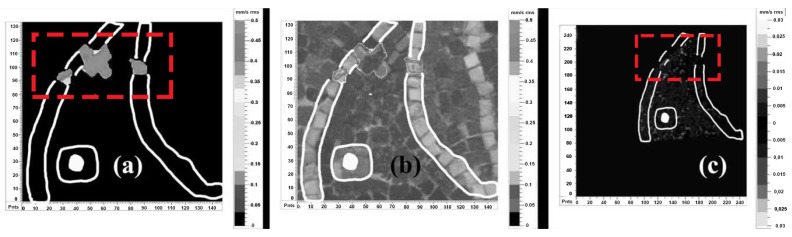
Scanning laser doppler vibrometry (SLDV) results in a custom-built mosaic model. Vibration maps of sample mosaic at 1525 Hz (a) a grayscale image representing the vibrational amplitude before restoration, the areas of detached tesserae show higher amplitude, corresponding to lighter shades of grey; (b) contour view before restoration; (c) amplitude after restoration, showing the more homogeneous distribution of amplitude values along the scanned surface. After Castellini et al. [76] (reprinted with copyright permission).
